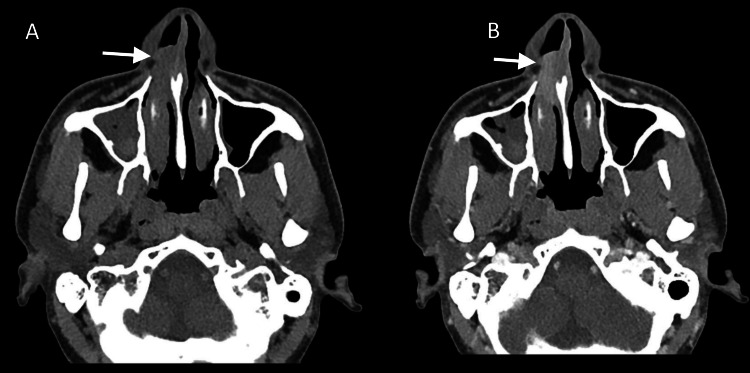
Axial views of non-contrast (A) and contrast-enhanced (B) CT PNS showing an enhancing soft tissue mass lesion in the right inferior nasal cavity (arrow) extending anteriorly into the vestibule. CT: computed tomography; PNS: paranasal sinus.
